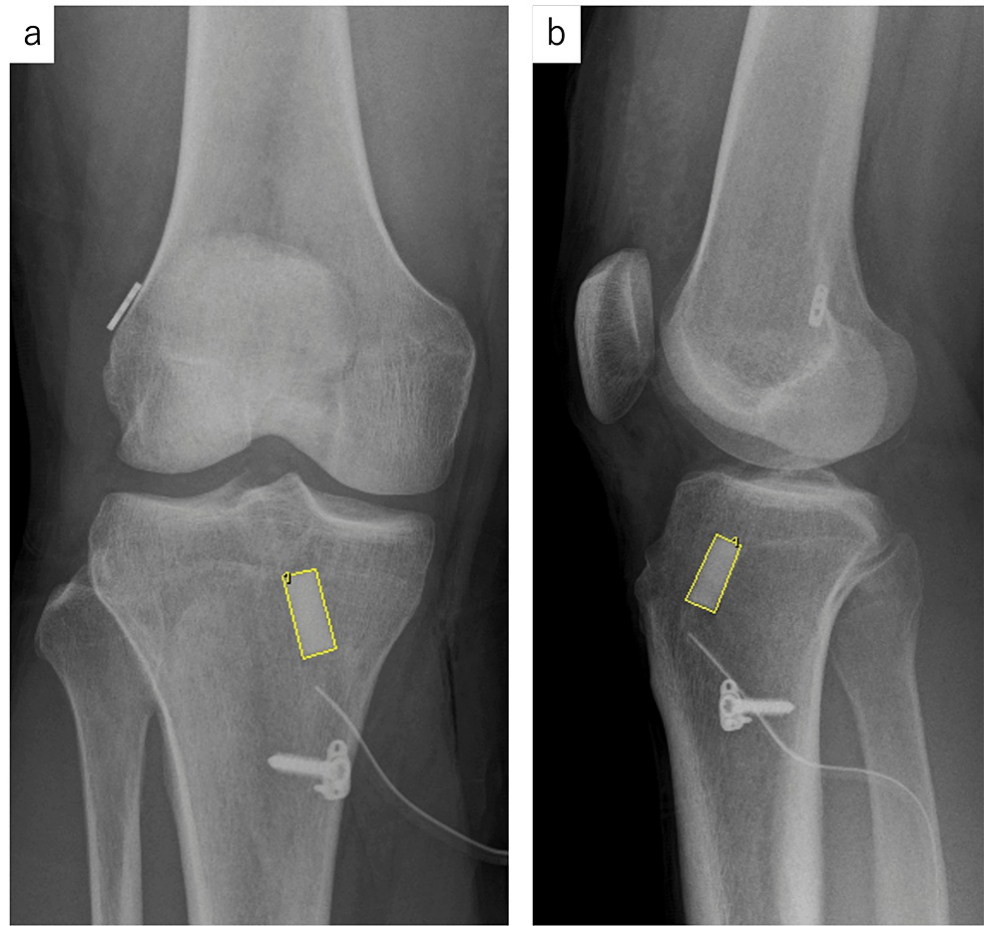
Measurement of the UDPTCP area (yellow rectangle) in (a) antero-posterior and (b) lateral views of radiograph
